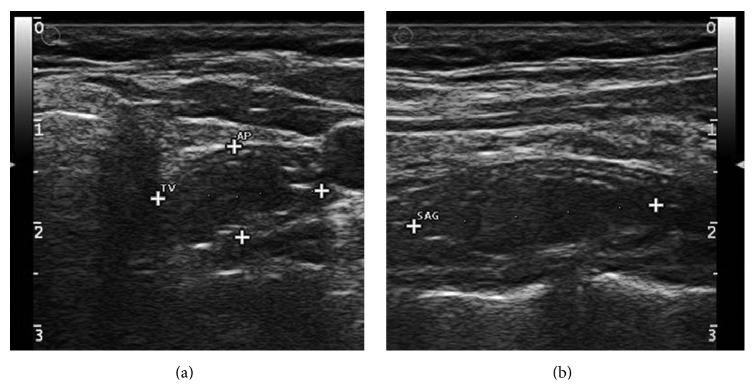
Transverse (a) and longitudinal (b) sonograms show a hypoechoic lesion with internal hyperechoic foci and hypoechoic rim.
